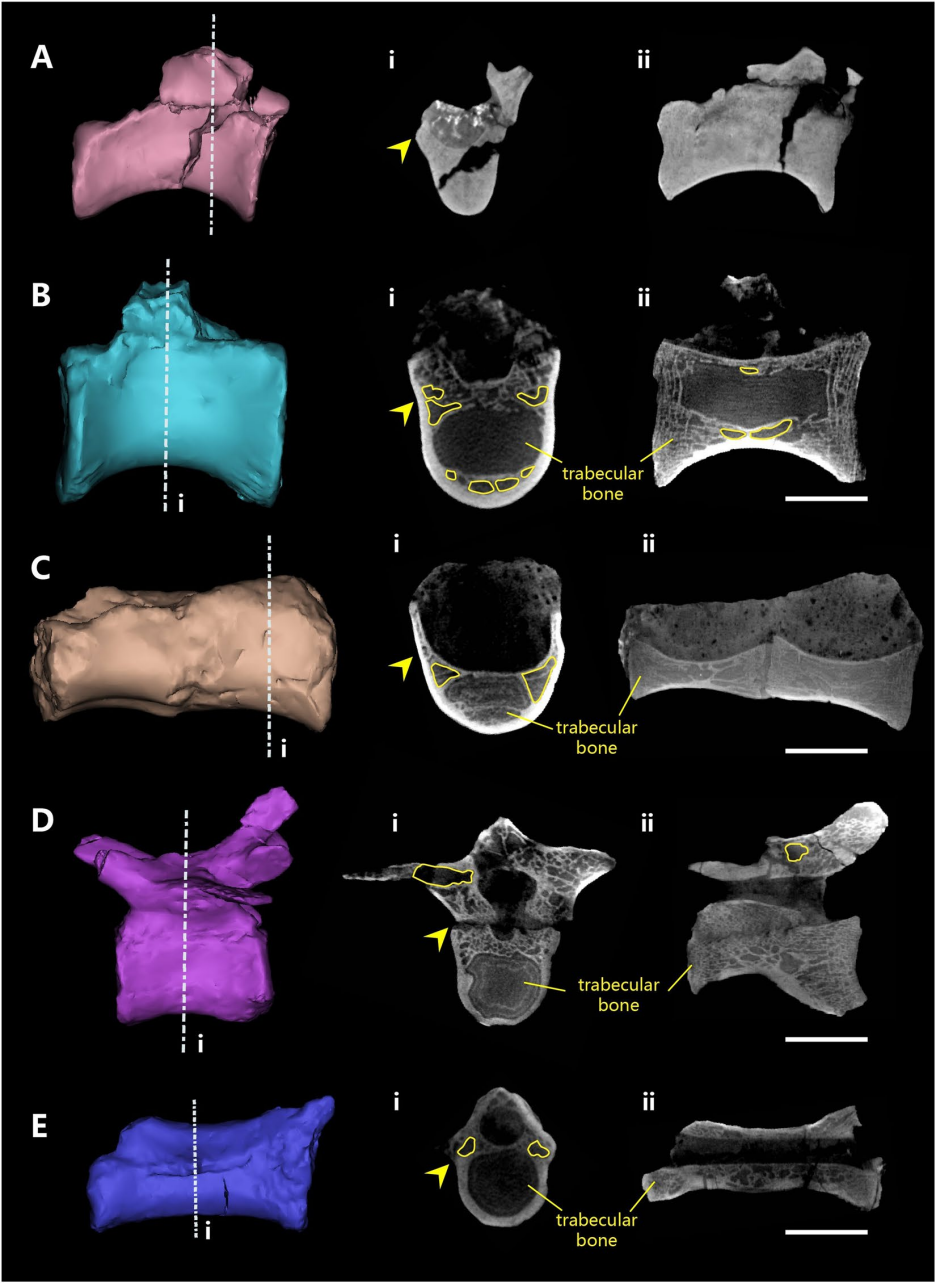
CT images of selected vertebrae of *Shishugounykus inexpectus* (IVPP V23567). (A), Cross section and midsagittal section of anterior dorsal vertebrae; (B), Cross section and midsagittal section of posterior dorsal vertebrae; (C), Cross section and midsagittal section of proximal sacral vertebrae; (D), Cross section and midsagittal section of proximal caudal vertebrae; (E), Cross section and midsagittal section of distal sacral vertebrae; pneumatic chambers are outlined in yellow; the sutures between centra and neural arches are marked by yellow arrows. (Scale bar, 10 mm).
